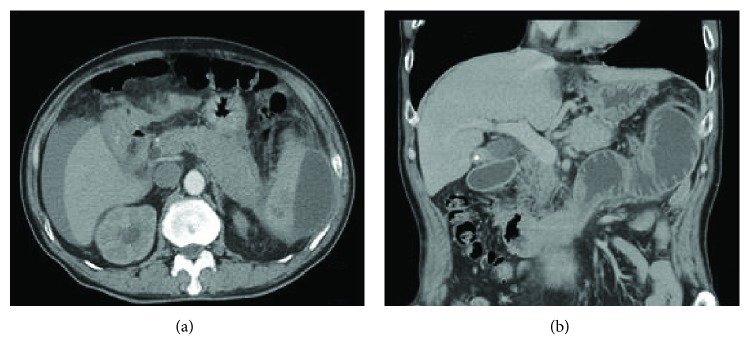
CT scan of patient no. 4. (a) Axial section of the CT scan showed a hematoma in the lower pole and lateral spleen area combined with the enlargement of the body and tail of the pancreas and free fluid around the liver and spleen. (b) Coronal section of the CT scan showed an obstruction of the small intestine.
